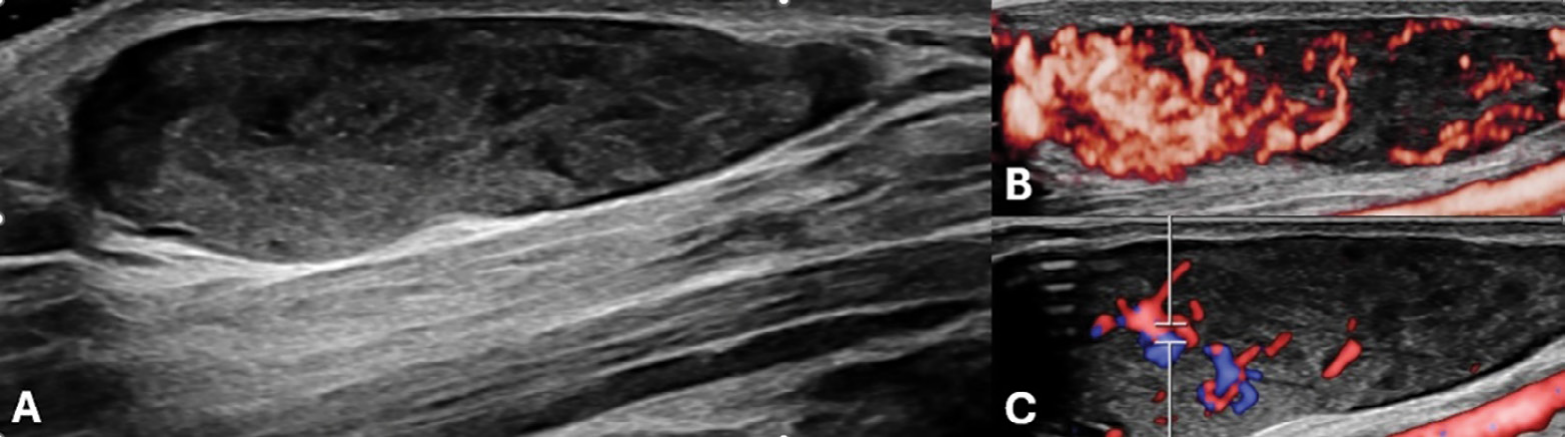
Doppler-Ultrasound: hypoechogenic lesion with tiny intralesional anechoic foci and extensive vascularization.
